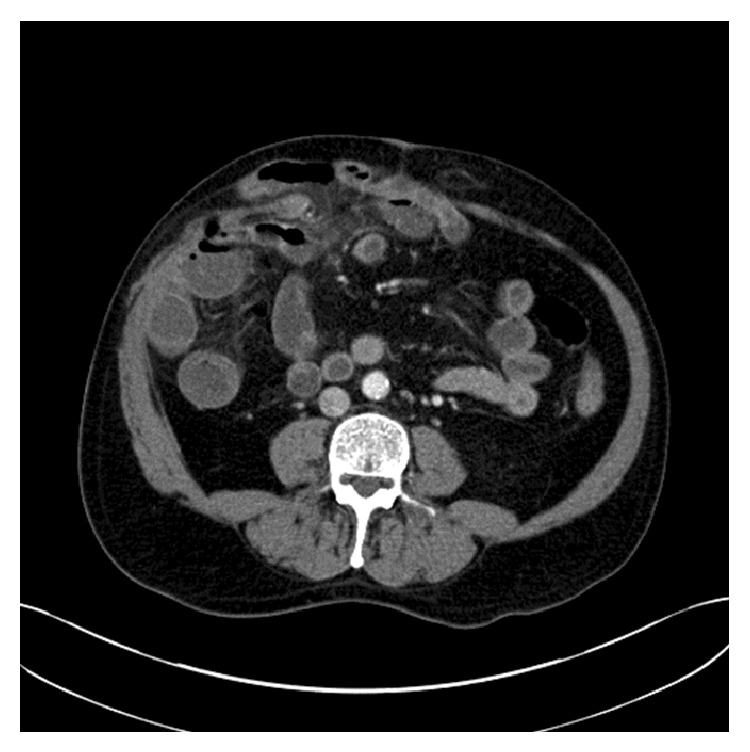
A CT image demonstrating the recurrent incisional hernia containing bowel loops.
